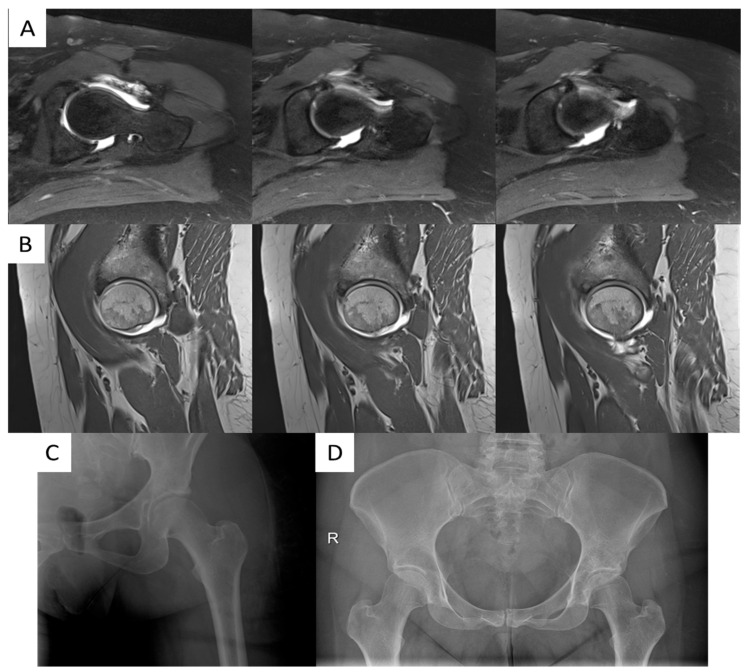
(A): preoperative T2-weighted MR arthrogram axial views showing antero-superior labral tear and acetabular subchondral bone cystic degeneration; (B): preoperative T1-weighted MR arthrogram sagittal views showing antero-superior labral tear and acetabular subchondral bone cystic degeneration; (C): immediate postoperative radiographic control; (D): 4-year radiographic follow-up. R: right.
